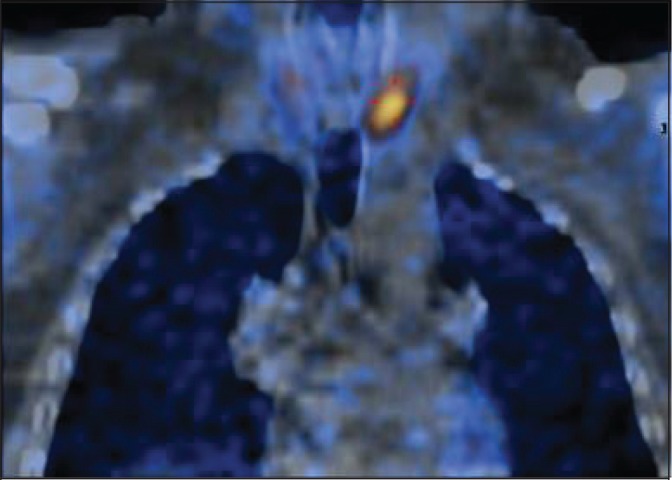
The single photon emission computed tomography showed an enlarged gland at the left side of the neck, just behind the inferior pole of the thyroid.
